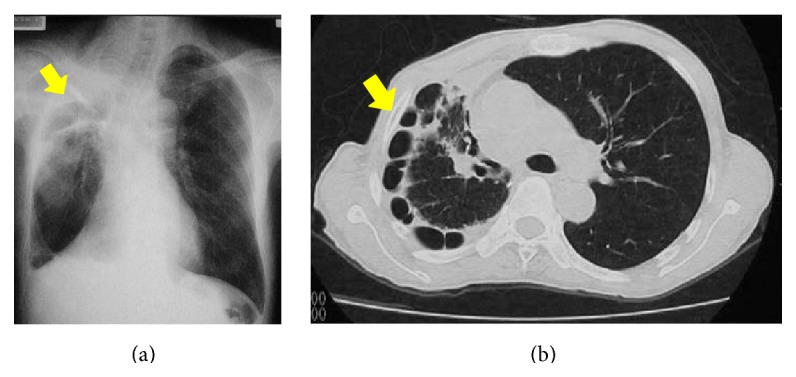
Chest X-ray (a) and chest contrast tomography scan (b) in Case 1. There are interstitial shadows and multiple cysts immediately below the pleura due to shrinkage of the lung, suggesting advanced destroyed lung.
